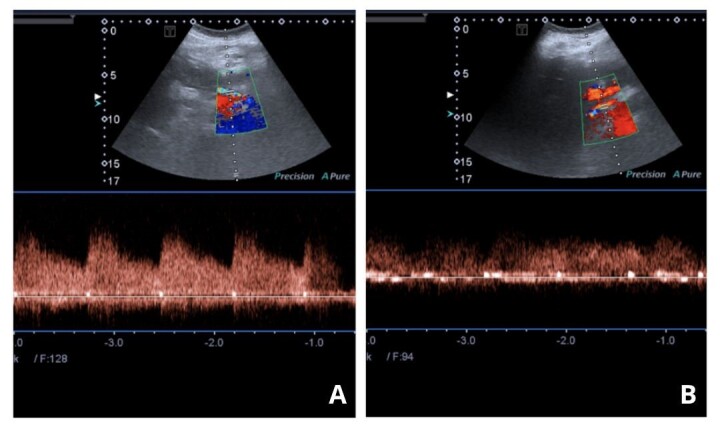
(A) Patent portal vein, with caliber maintained and Doppler velocimetry pattern preserved; (B) Surgical graft communicating between gastroduodenal and right hepatic arteries, with hepatopetal flow, no signs of turbulence, and low resistance peak systolic velocity (VPS) of 120 cm/s. Authors’ files.
